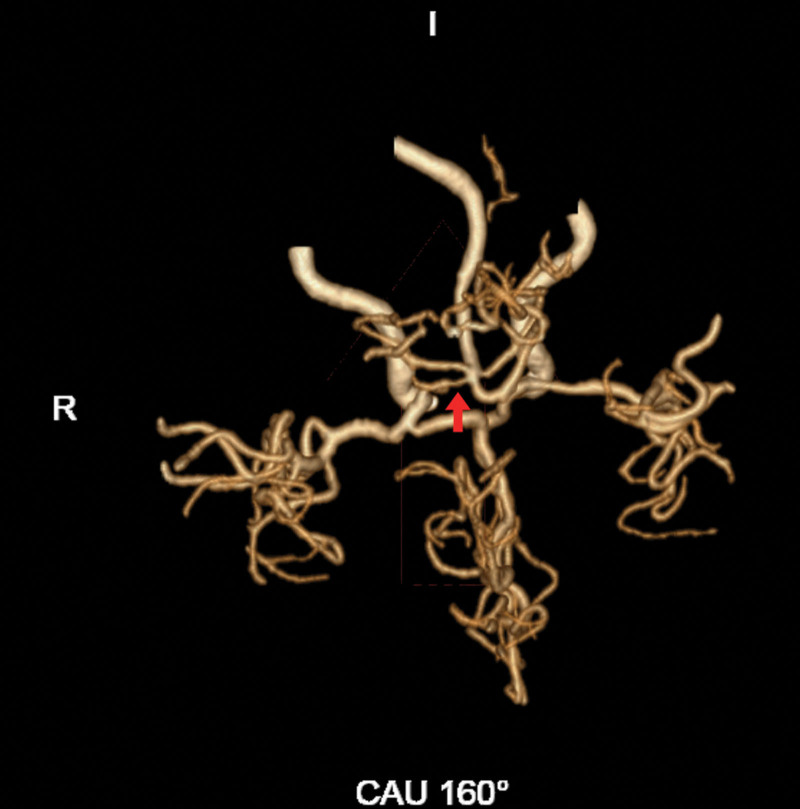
Cerebral CTA performed on the 1st day of neurological deterioration (day 2 post-injury). Cerebral CTA reveals severe stenosis of the right PCA at the P1 segment and complete occlusion at the P2 segment. An arrow indicates the site of the P1 segment stenosis. This vascular finding was deemed an incidental, chronic preexisting condition, as the distribution of the acute cerebral infarcts was inconsistent with the vascular territory supplied by the right PCA. CTA = computed tomography angiography, PCA = posterior cerebral artery.
